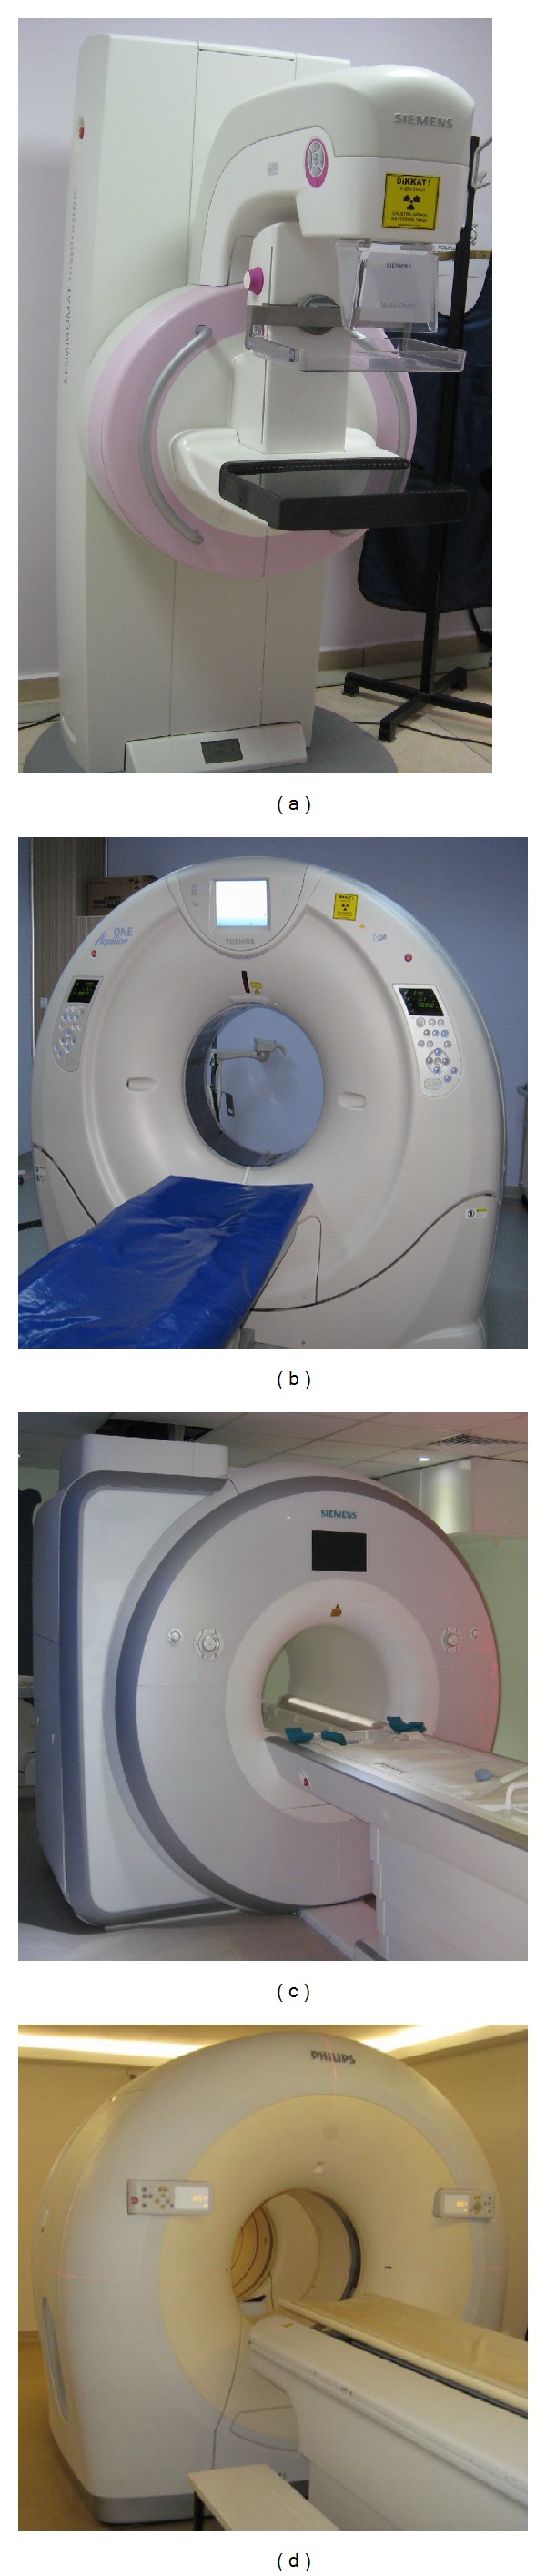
Clinical radiology systems: (a) tomosythesis, (b) computer tomography, (c) magnetic resonance imaging, and (d) positron emission tomography/computer tomography used in this study.
